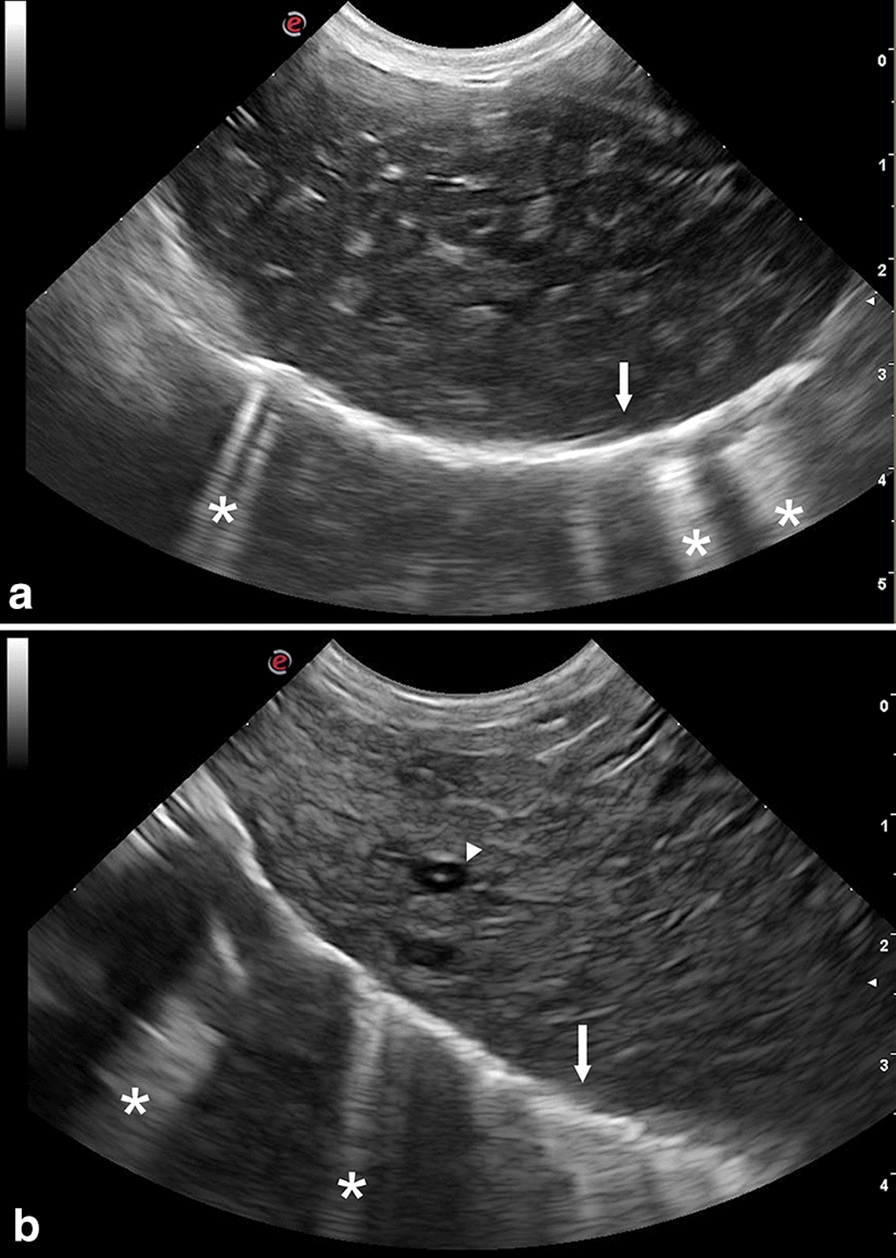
Ultrasonographic appearance of the lamb’s liver affected by acute cysticercosis. a Ultrasonographic transversal section of the liver showing a diffusely heterogeneous parenchyma crossed by hypoechoic irregular tracts. The diaphragmatic surface of the liver appears irregular, thickened and hyperechoic (arrow). The lung surface presents several B-lines (asterisks). b Ultrasonographic longitudinal section of the liver showing a diffusely heterogeneous parenchyma in which intraparenchymal cysts are visible (arrowhead). The diaphragmatic surface of the liver appears irregular, thickened and hyperechoic (arrow). The lung surface presents several B-lines (asterisks)
